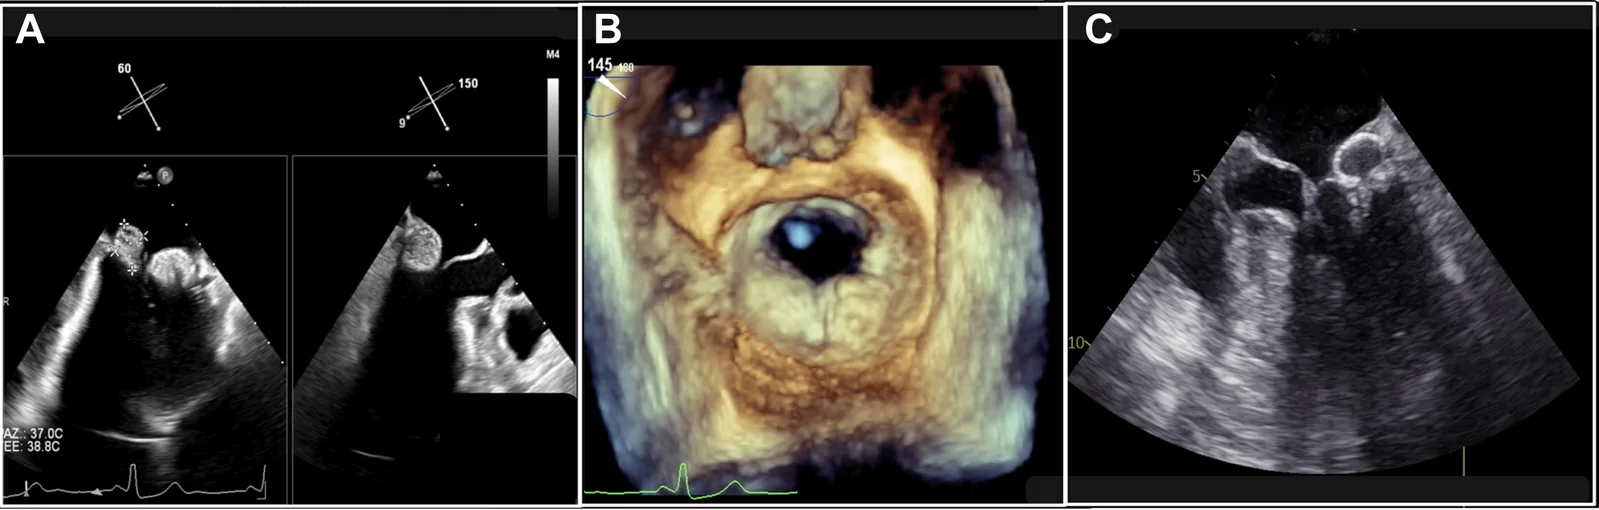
Transesophageal Echocardiography (A) X-plane TEE view of CMAC before vacuolization; (B) 3D TEE view of CMAC; (C) TEE view of CMAC after vacuolization. CMAC = caseous mitral annular calcification; TEE = transesophageal echocardiography.
